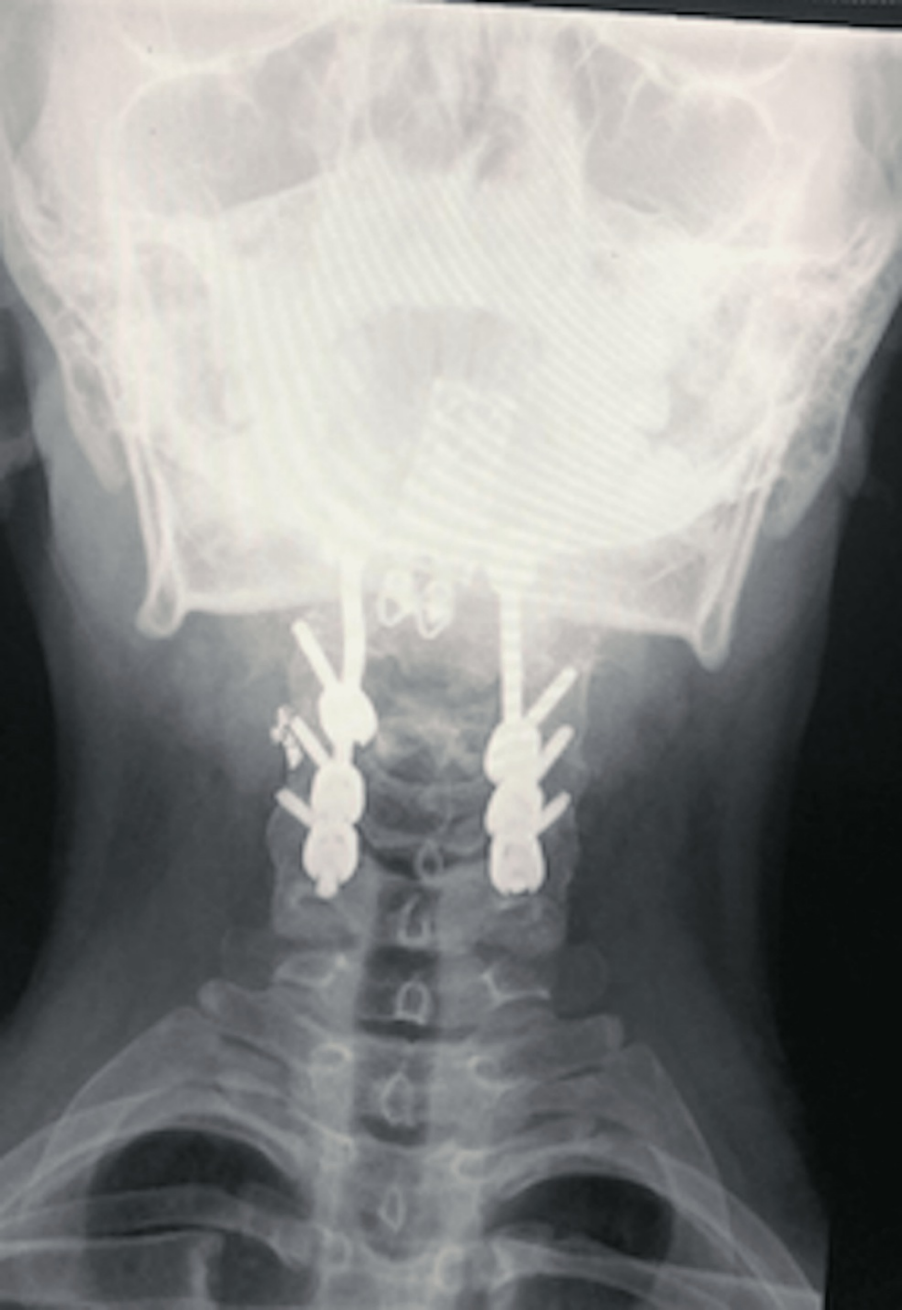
Post-operative anteroposterior view X-ray The posterior fixation is from occiput to C5 using lateral mass screws.
